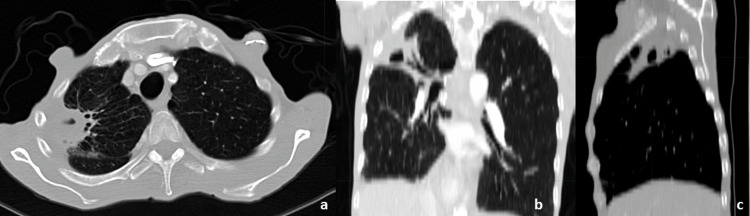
One-month post-radiation treatment follow-up CT scan. a, b, and c: axial, coronal, and sagittal image planes CT: computed tomography
